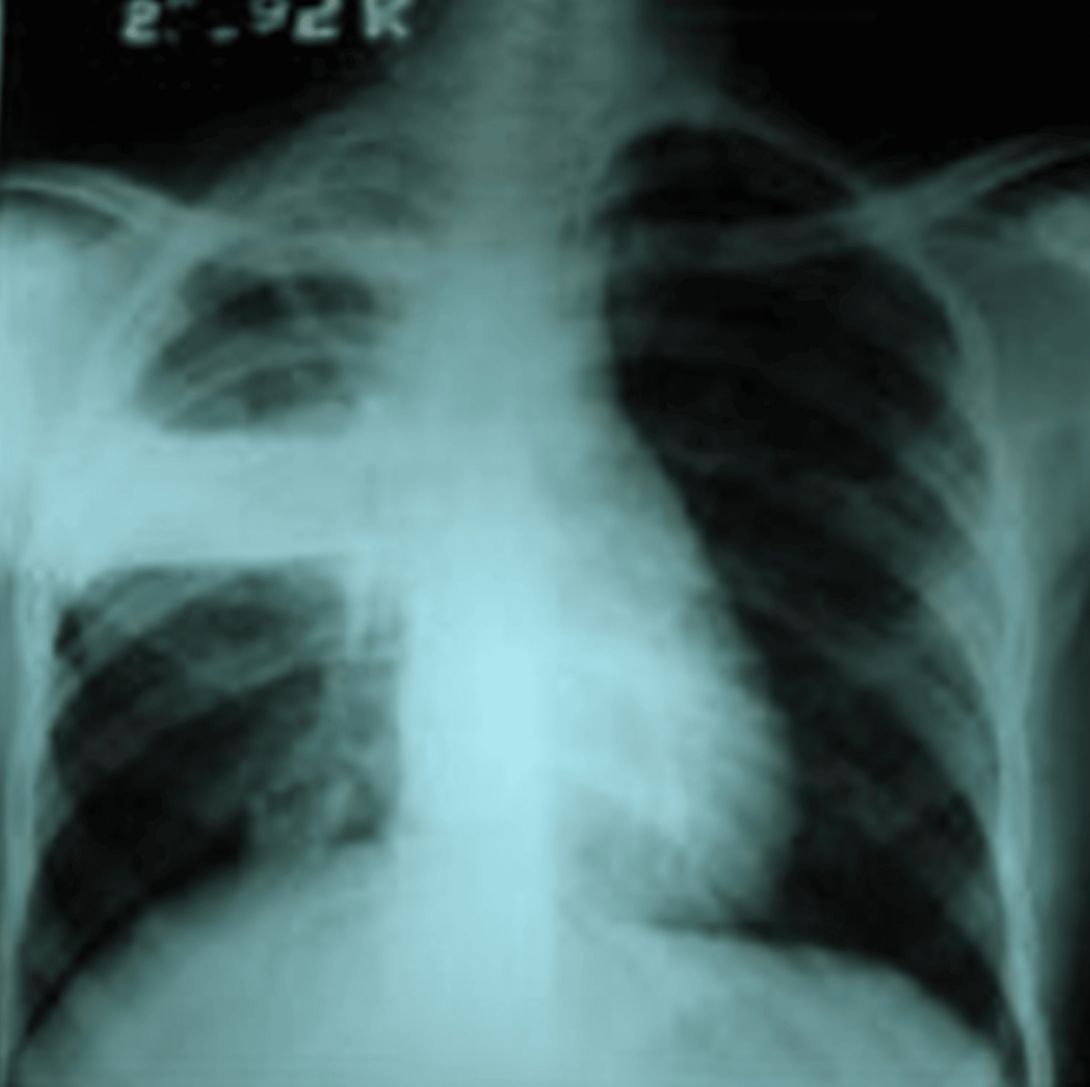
Chest radiograph showing right upper lobe cavitary lesion. Posteroanterior chest X-ray demonstrating a well-defined cavity with an internal air-fluid level in the right upper lobe, without associated fibrotic changes.
